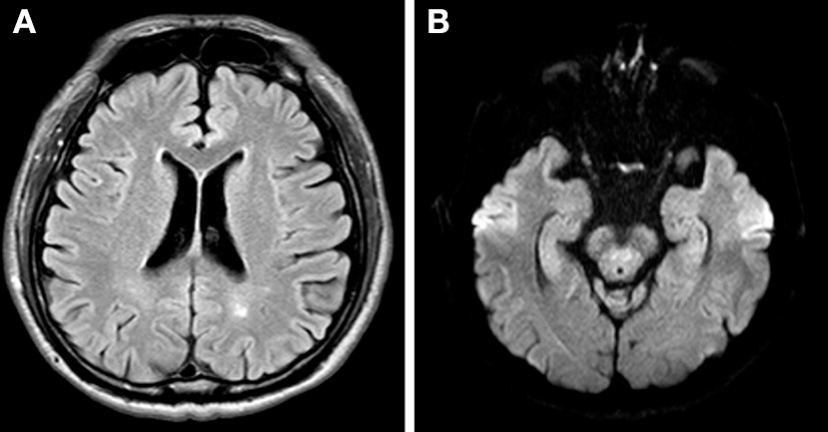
(A) Axial view of T2 MRI at lateral ventricle slice showed no obvious inflammatory lesion along frontoparietal cortex (B) Axial view of T2 MRI at the midbrain slice showed no obvious inflammatory change at oculomotor nerve.
